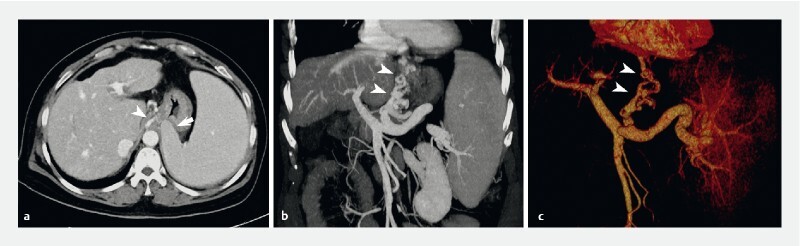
Computed tomography venography of the portal vein system showing the esophageal varices extending below the cardia into the lesser curvature of the stomach (arrowheads), rather than the fundus, with the enlarged spleen seen to be compressing the gastric fundus in image
